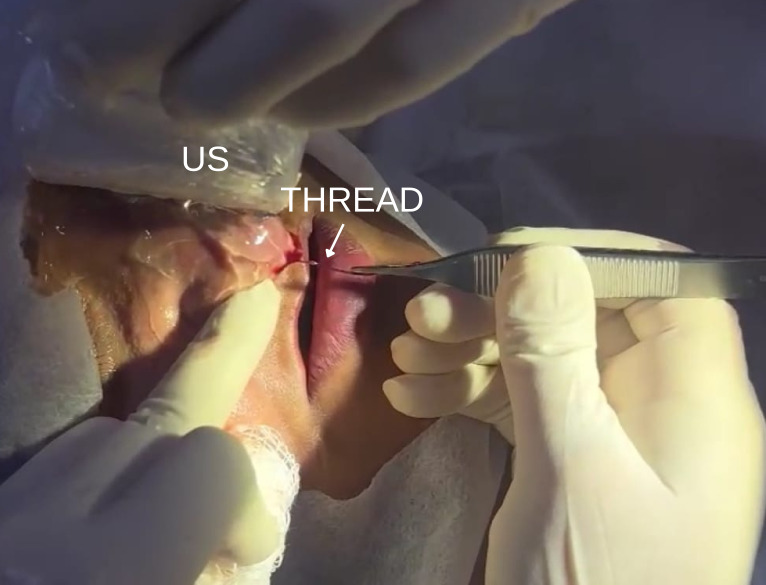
Intraoperative view of nasal incisions with removal of PDO thread using surgical forceps under point of care ultrasound (POCUS) guidance. US, ultrasound.
